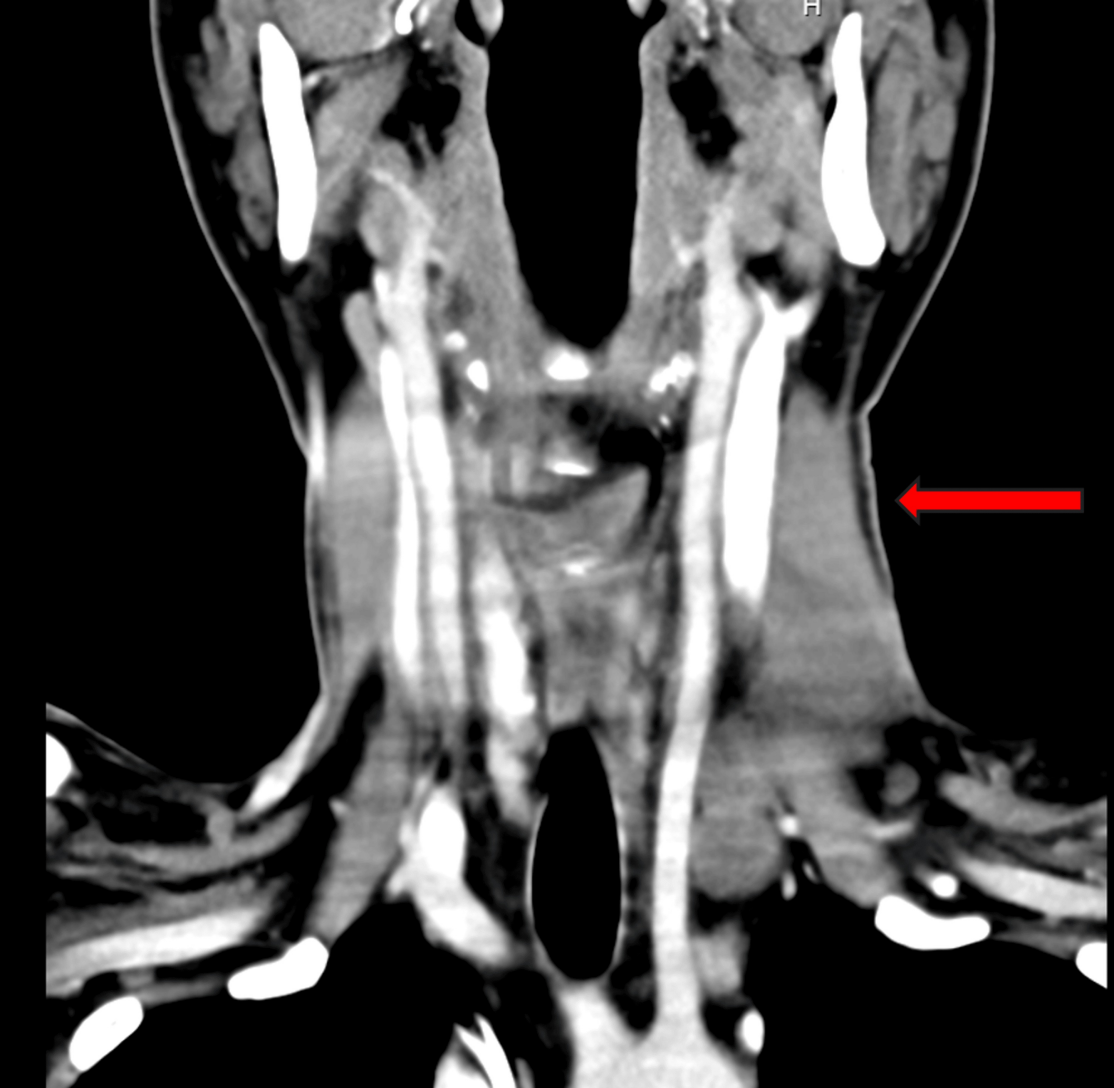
Interval imaging of the CT neck showing marked decrease in the size and number of left jugulodigastric chain adenopathy as labeled by the red arrow, largest measuring 13 mm x 24 mm x 38 mm
